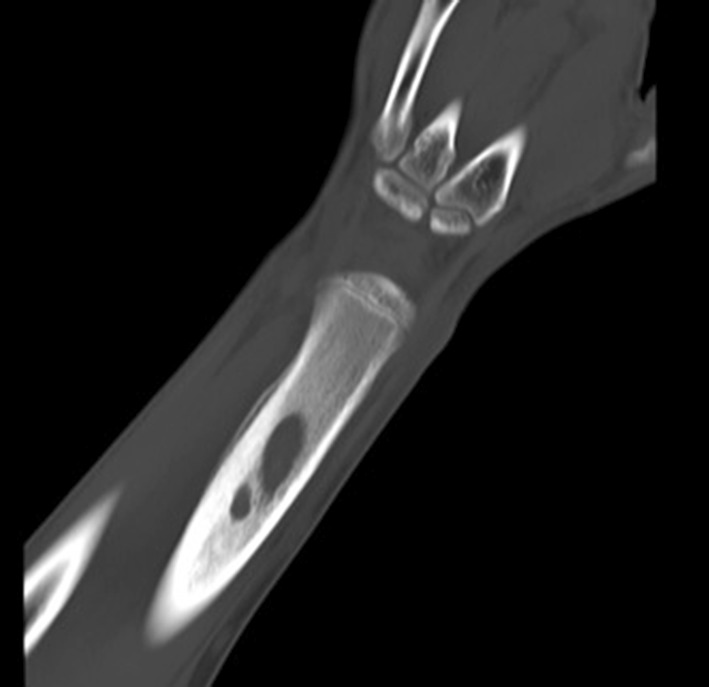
CT scan of the *left* distal radius confirming the lucent area with cortical thickening
